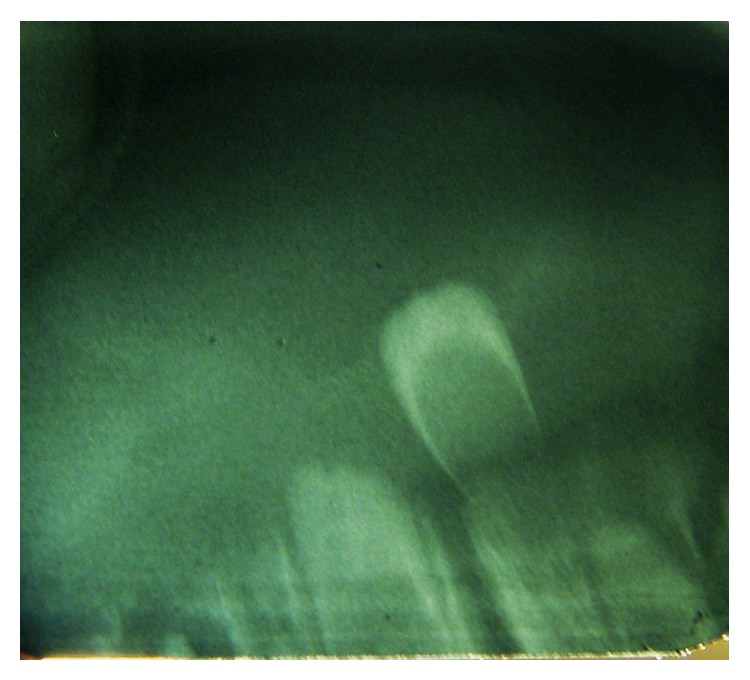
Periapical radiograph showing the natal tooth in the mandibular anterior area.
